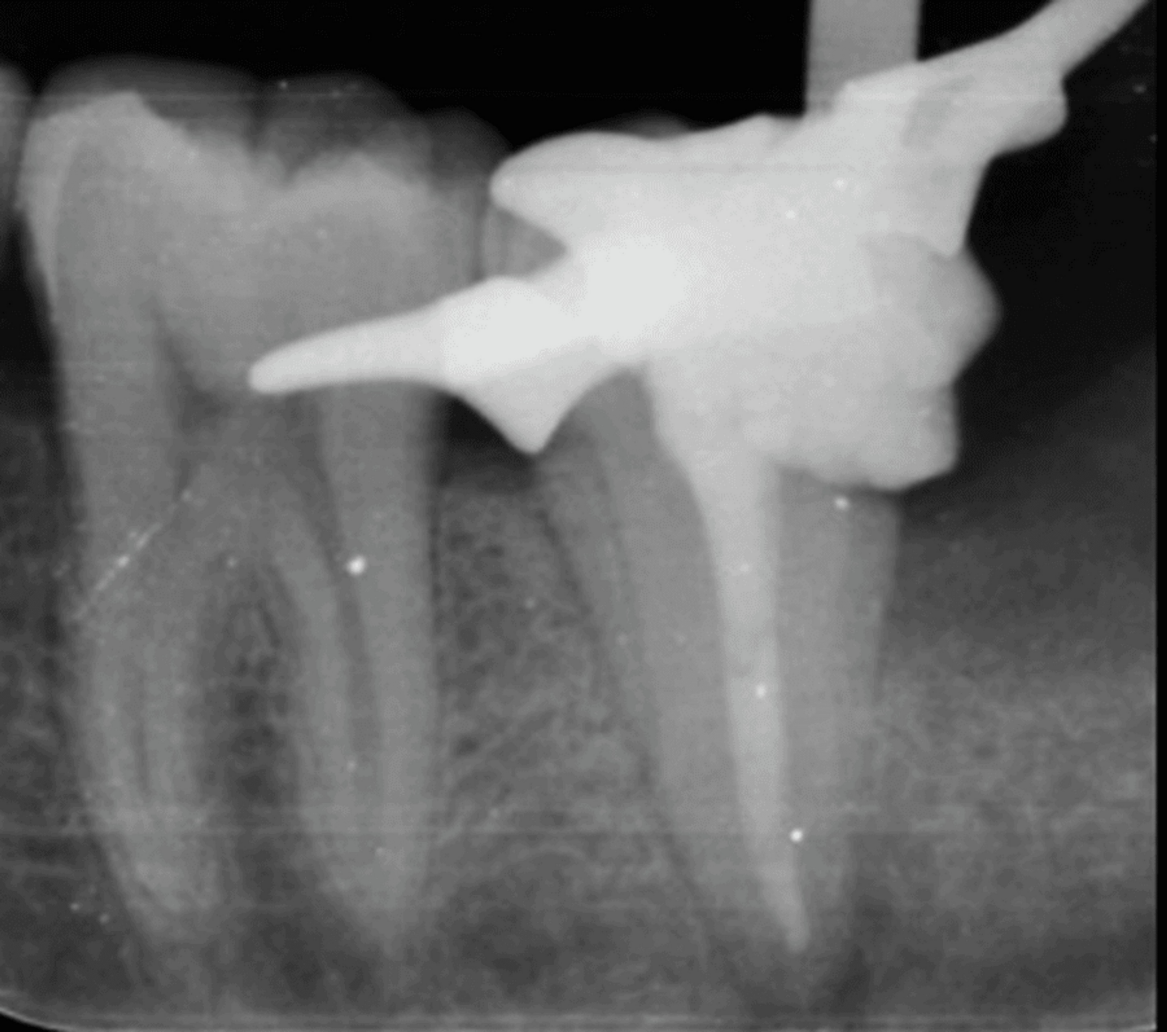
Intraoral periapical radiograph showing obturation with 47 This intraoral periapical radiograph shows obturation with 47
